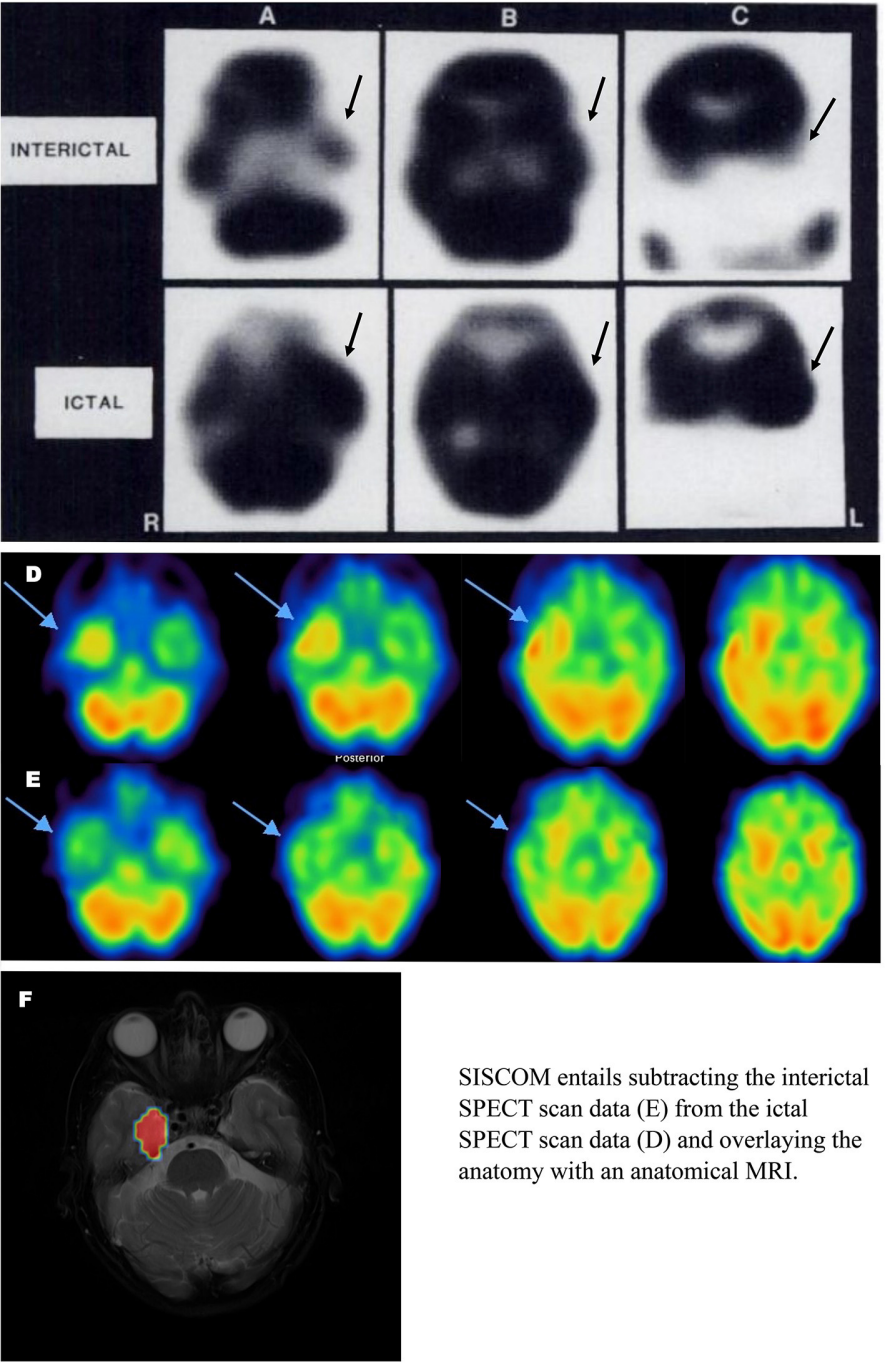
Ictal and Interictal SPECT (A–C) Example of SPECT scan studies cited in TTASAAN report. Perfusion tracer is iodinated N,N,N'-trimethyl-N'-(2-hydroxy-3– methyl-5-iodobenzyl)-1,3-propane-diamine (HIPDM). Anatomical details are lacking [The figure was originally published in JNM. © SNMMI (149)]. (D,E) Modern 2021 HMPAO SPECT perfusion scans for seizure localization. (D) Ictal scan. (E) Interictal scan. (F) SISCOM image wherein interictal scan data is subtracted from ictal scan data and the resulting data is overlayed on an anatomical MRI. Images courtesy of– Leonard Numerow MD FRCP(C), Radiology and Nuclear Medicine, Cumming School of Medicine, University of Calgary, Calgary, Alberta, Canada.
